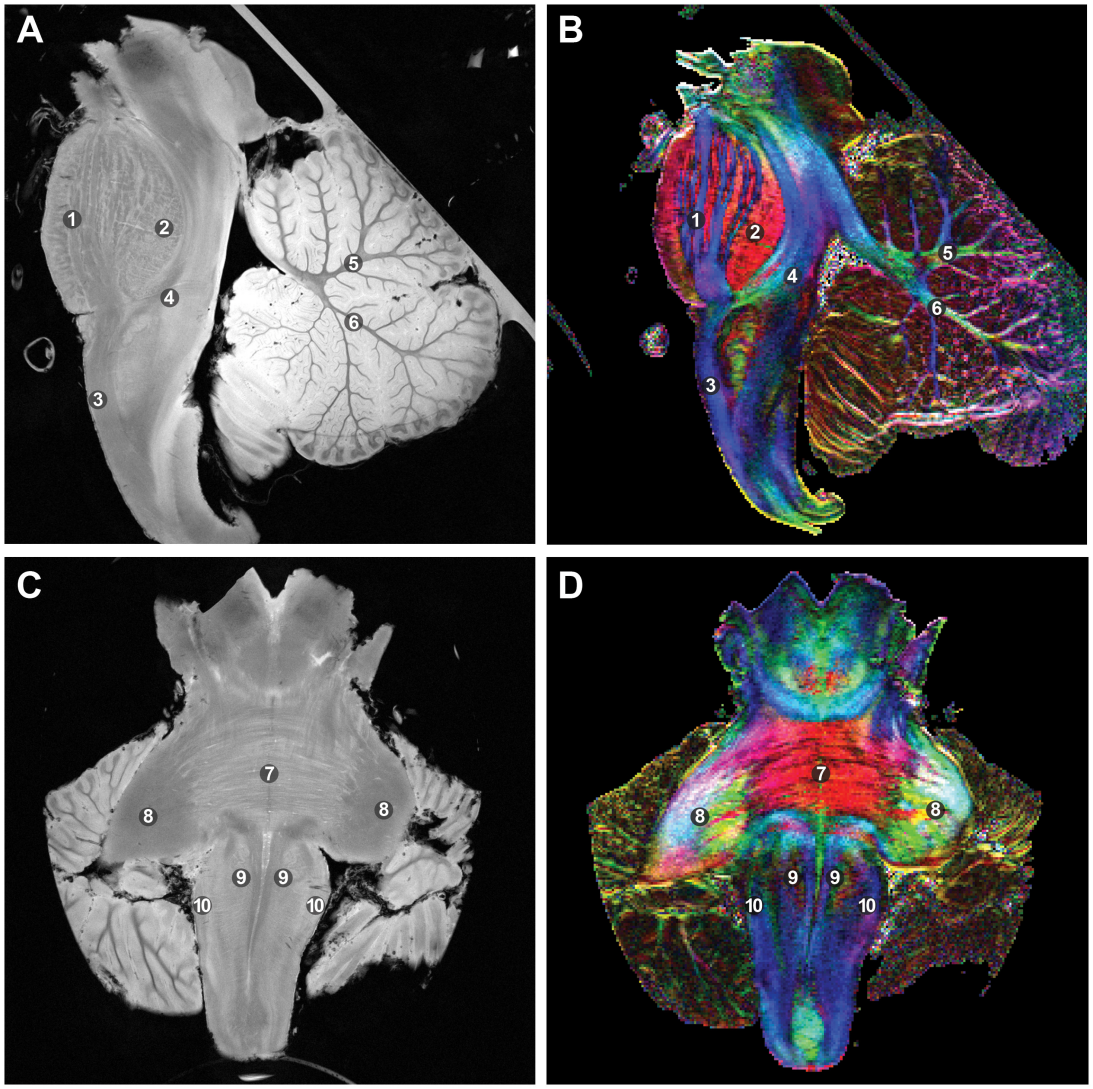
T2-weighted fast low-angle shot sequence MR images with an in-plane resolution of 0.55 mm and fractional anisotropy (FA) color mapping, which delineates and separates crucial structures. The coloration in the FA map corresponds to local tract direction: red denotes left-right, blue signifies inferior-superior, and green indicates anterior-posterior. (A) Mid-sagittal view. (B) Corresponding fractional anisotropy color mapping. (C) Mid-coronal view. (D) Corresponding color mapping. 1: Longitudinal fasciculus pons. 2: Transverse fasciculus pons. 3: Pyramidal tract. 4: Medical lemniscus. 5: Apical arm of the cerebellar arbor vitae. 6: Central arm of the cerebellar arbor vitae. 7: Pontocerebellar fibers. 8: Brachium pontis. 9: Pyramids. 10: Olive. *Used with permission from Barrow Neurological Institute, Phoenix, Arizona*.
